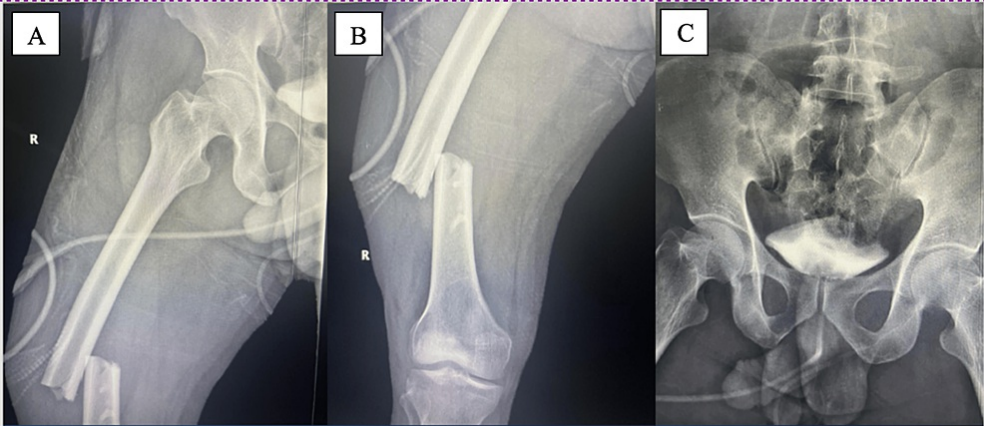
Preoperative radiographs of the right femur, revealing a closed femoral shaft fracture. Anteroposterior view (A and B); anteroposterior view of the pelvis (C).
